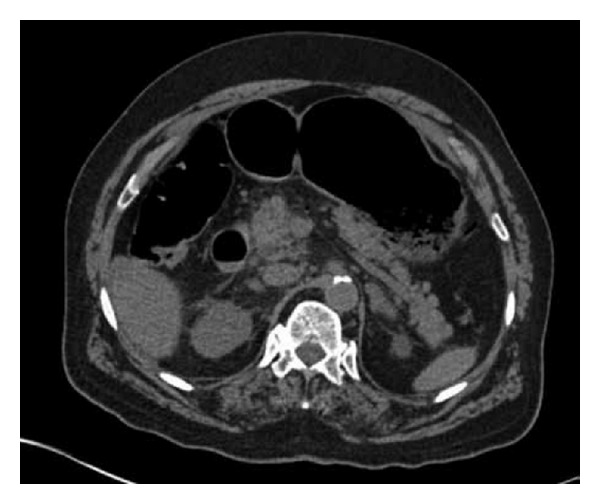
CT showing a calcified plaque at the origin of the celiac axis (Case 1).
